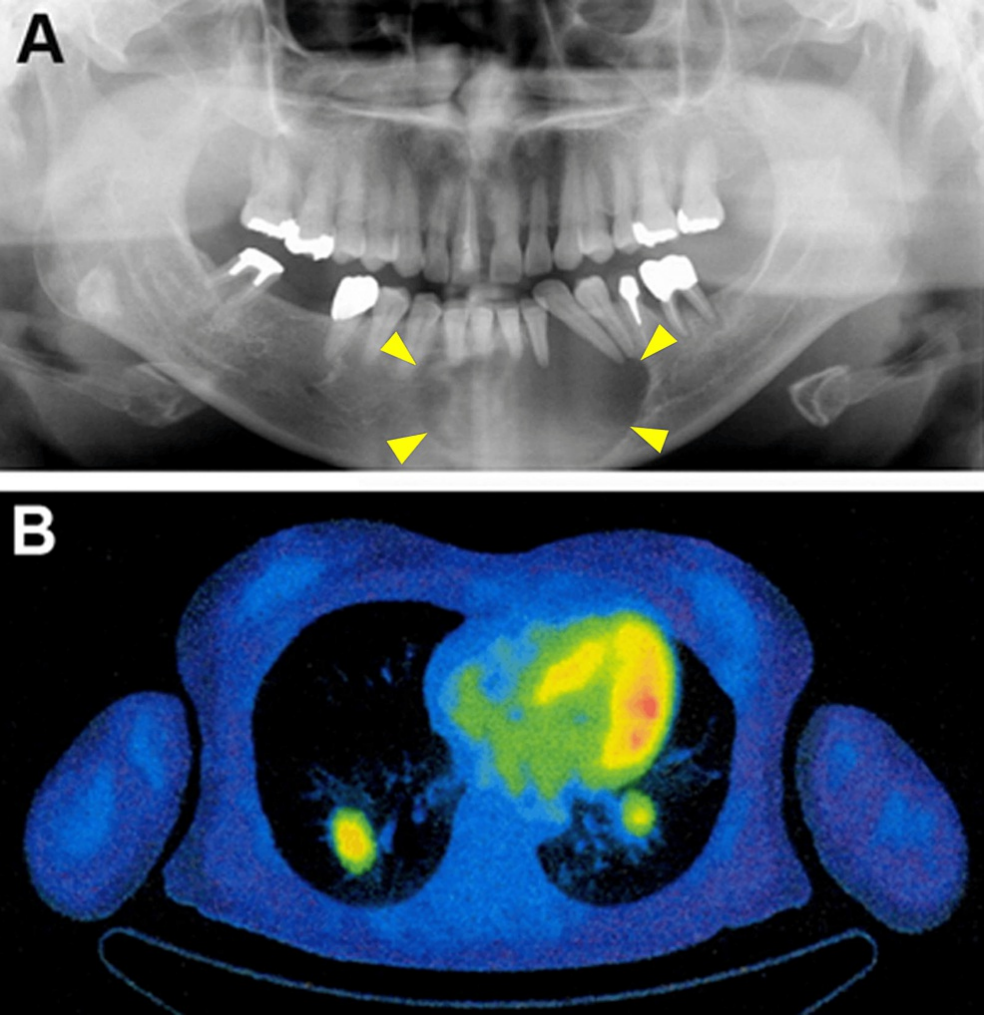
Panoramic X-ray image at the initial administration and 18FDG-PET/CT image four years later (A) The panoramic radiograph at the first visit shows a well-demarcated radiolucent lesion (arrowheads) in the lower anterior mandible; (B) An 18FDG-PET/CT image displays increased uptake in the bilateral lung nodules four years after mandibulectomy 18FDG: fludeoxyglucose F18; PET: positron emission tomography
